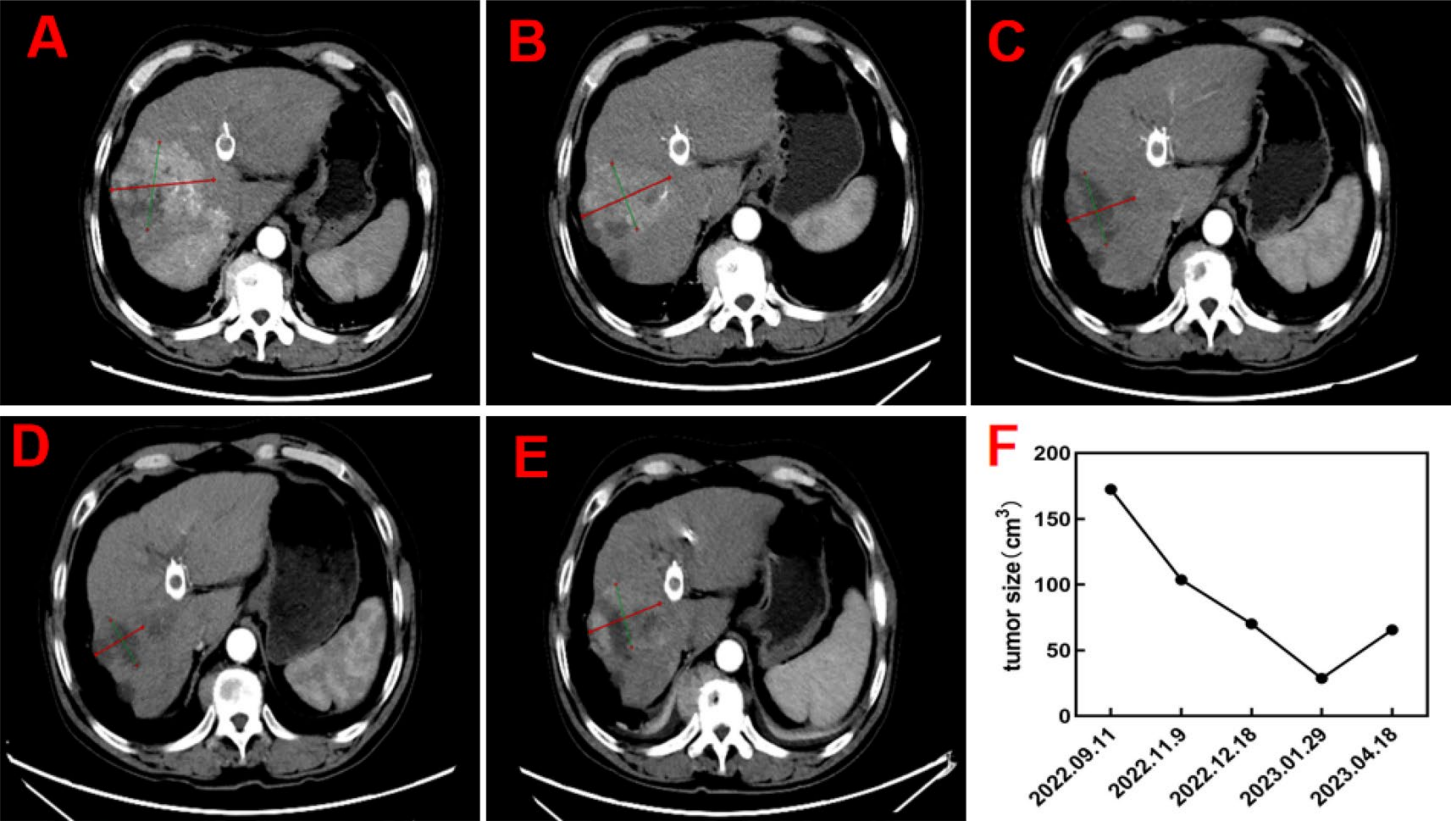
The change size of tumor before and after TACE treatment: CT scans showed pronounced enhancement in the tumor area pre-TACE (A), which weakened or disappeared post-TACE treatment (B, C, D), and reappeared after 4 months (E). The tumor size changes pre- and post-treatment were shown in panel F
